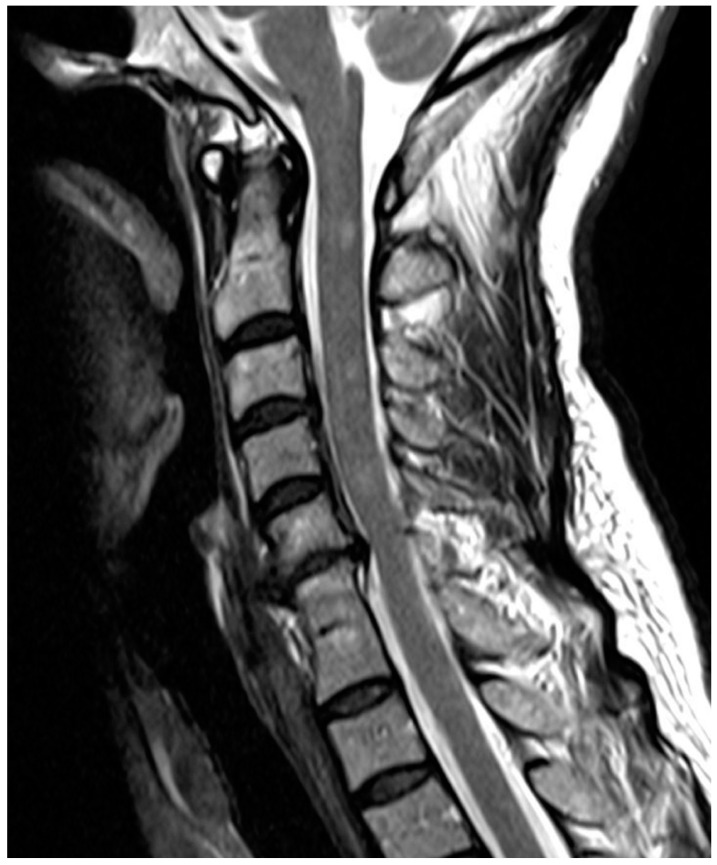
Sagittal T2-weighted fast spin-echo sequence; 53 y/o female; congenital block vertebra C6/7 with degenerative changes in segment C5/6 (disk prolapse) and otherwise normal cervical spine consistent with ASDI. Please also note T2-hyperintense lesions in the cervical spinal cord consistent with demyelination in this multiple sclerosis patient.
